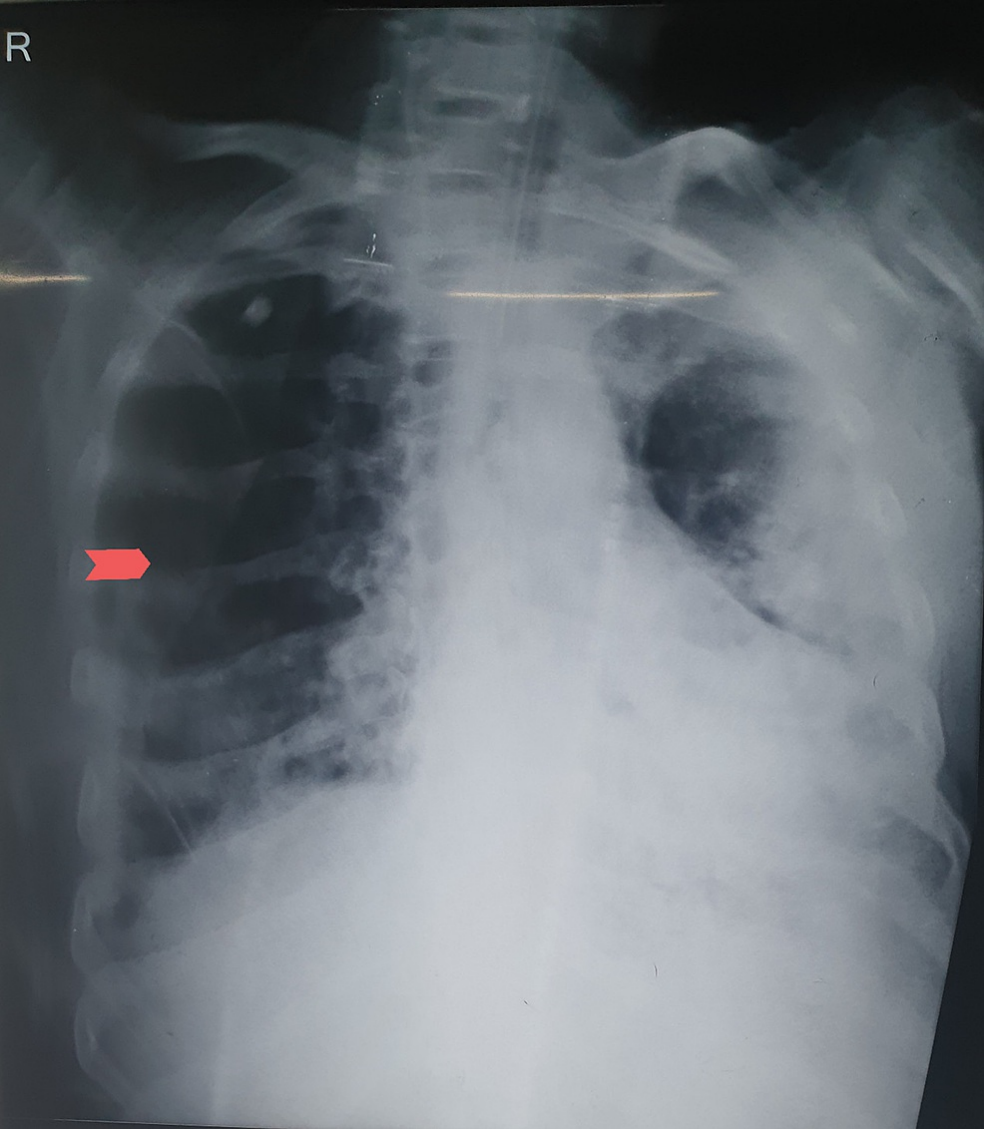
CXR demonstrating right-sided pneumothorax (shown by arrow). CXR, Chest X-ray
